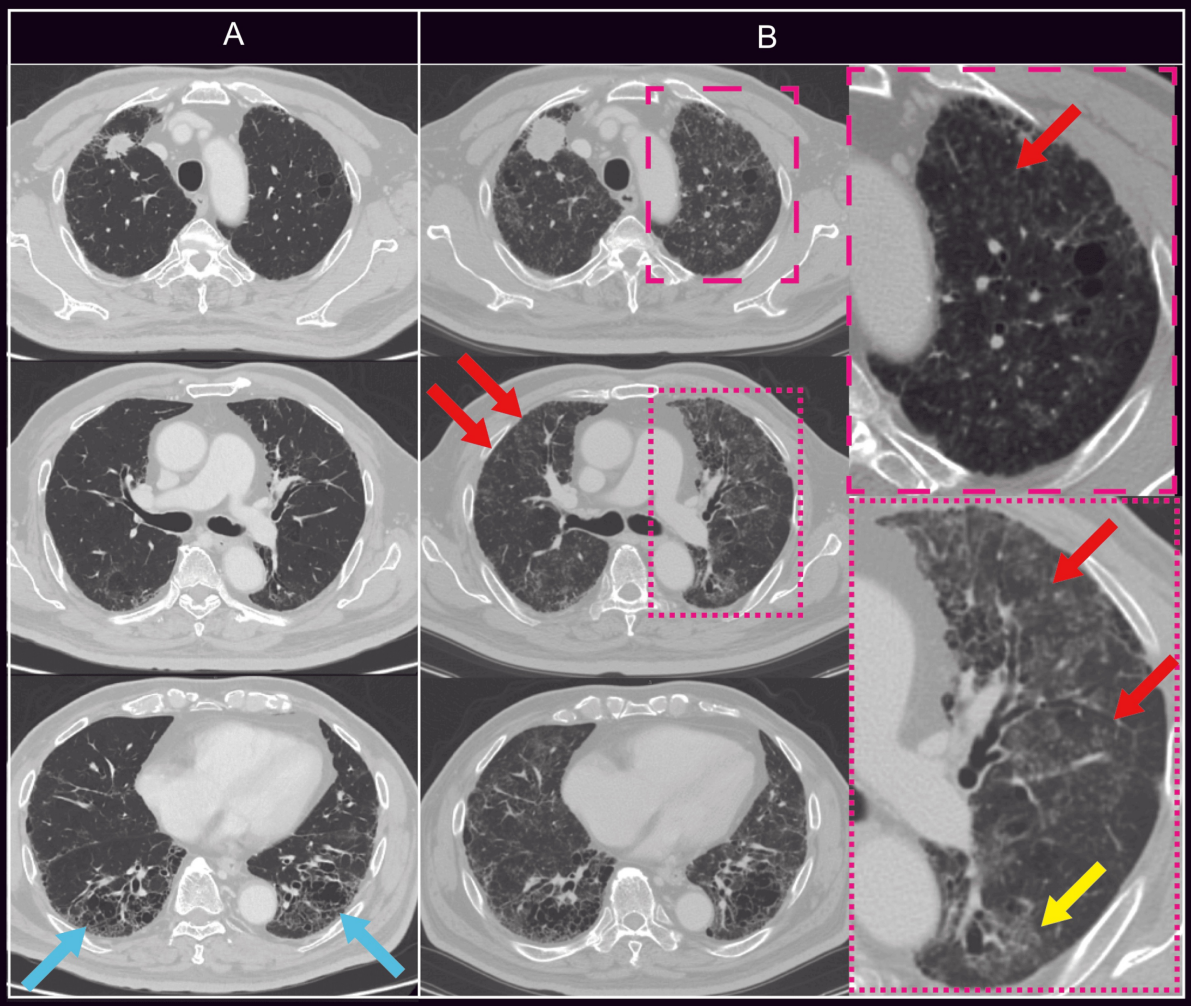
Chest CT images obtained before initiation of UFT/LV plus bevacizumab (A) and three months after treatment initiation, at the onset of dyspnea (B) Before treatment initiation, fibrosis and honeycombing were observed in both lower lung fields (blue arrows). After the onset of dyspnea, newly developed centrilobular nodules (red arrows) and ground-glass opacities (yellow arrows) were noted in both lungs. UFT: tegafur–uracil; LV: leucovorin
